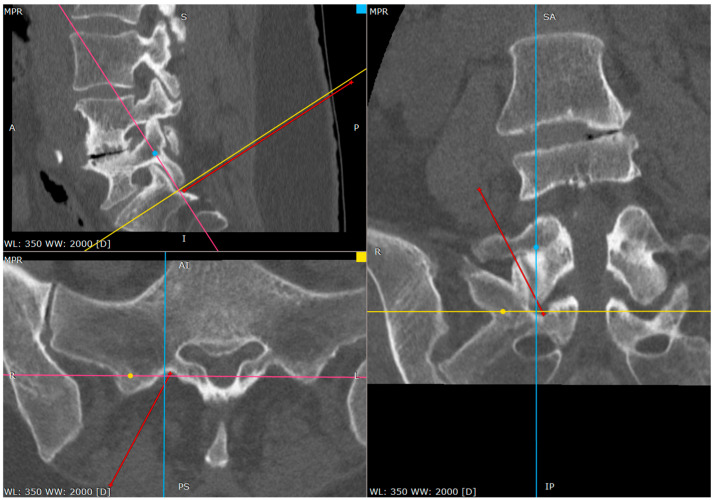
Needle against inferior part of S1 facet surface (CT tri-plane reconstruction).
